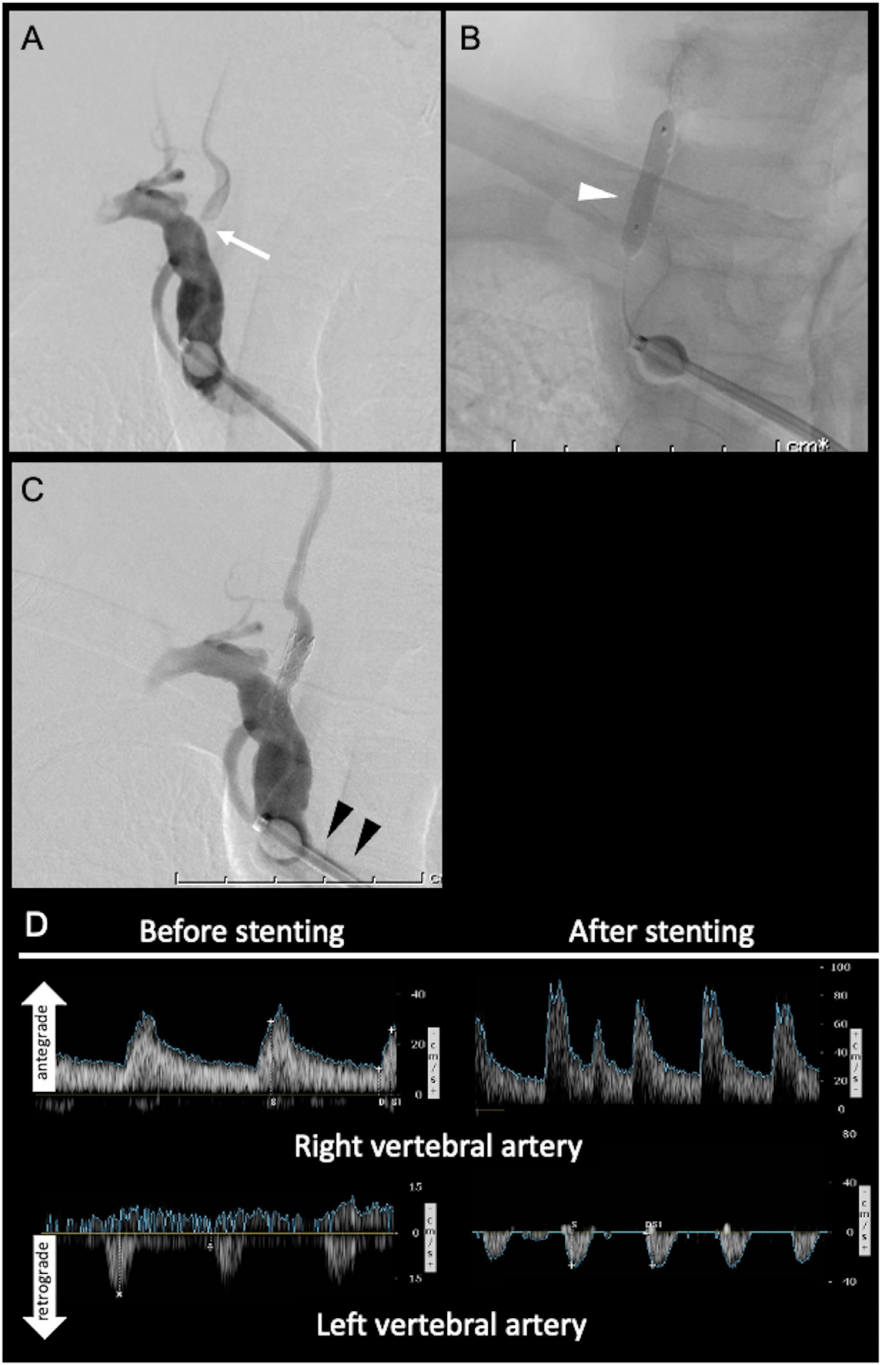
(A) Right subclavian artery injection demonstrates severe stenosis of the right vertebral artery (white arrow) and retrograde filling of the subclavian artery through the partially inflated balloon (arrowheads). B) Non-subtracted image shows non-compliant balloon inflated over the stenotic lesion (white arrowhead). (C) Right subclavian artery injection shows sufficient dilatation of the right vertebral artery. Note that retrograde filling was not observed because of the balloon in the subclavian artery (black arrowheads). (D) Doppler ultrasonography of the right and left vertebral artery obtained before and after the stenting showing increase in antegrade flow of the right vertebral artery.
